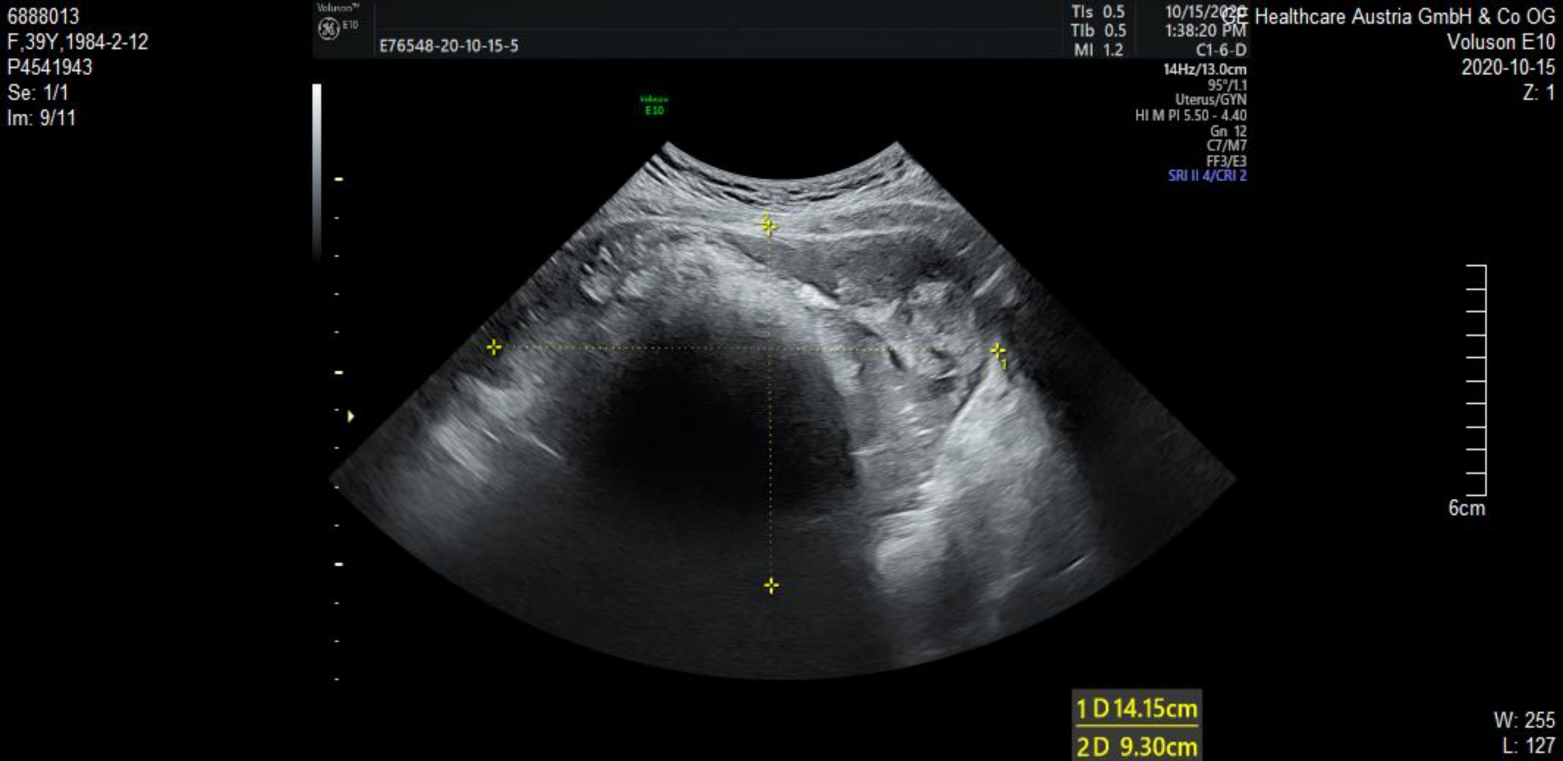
A pelvic mass examined by transvaginal ultrasound. Uneven echo mass of 14.2 cm × 9.3 cm × 12.8 cm was observed in the pelvic cavity, the boundary was clear, attenuation was observed in the rear, and no blood flow signal was observed.
